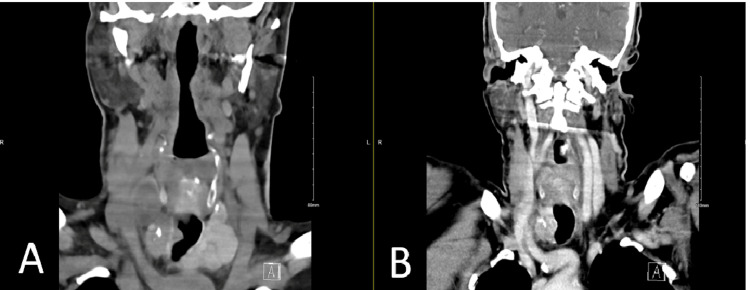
CT scan showing tracheal invasion. (A) CT scan showing preoperative right tracheal invasion. (B) CT scan showing the remaining postoperative right tracheal invasion.
